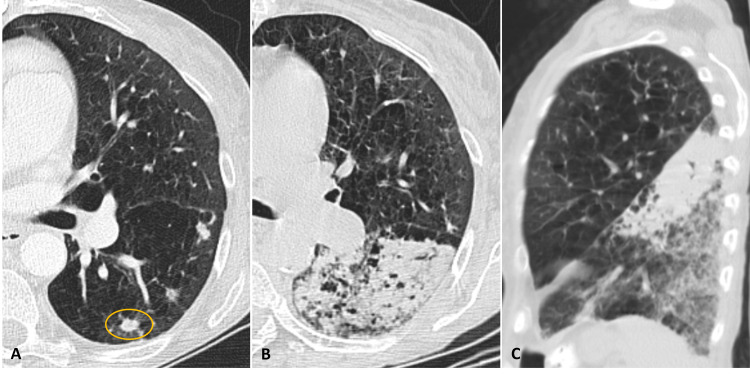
Pulmonary nodule and alveolar haemorrhage. Pulmonary window CT images. (A) Axial plane showing the subpleural nodule (circle) in the posterior segment of the inferior left pulmonary lobe before the biopsy. (B) Axial plane the day after the biopsy demonstrating the large alveolar haemorrhage. (C) Coronal reformation allows better evaluation of the extent of the alveolar haemorrhage, occupying almost the entire left lower lobe.
